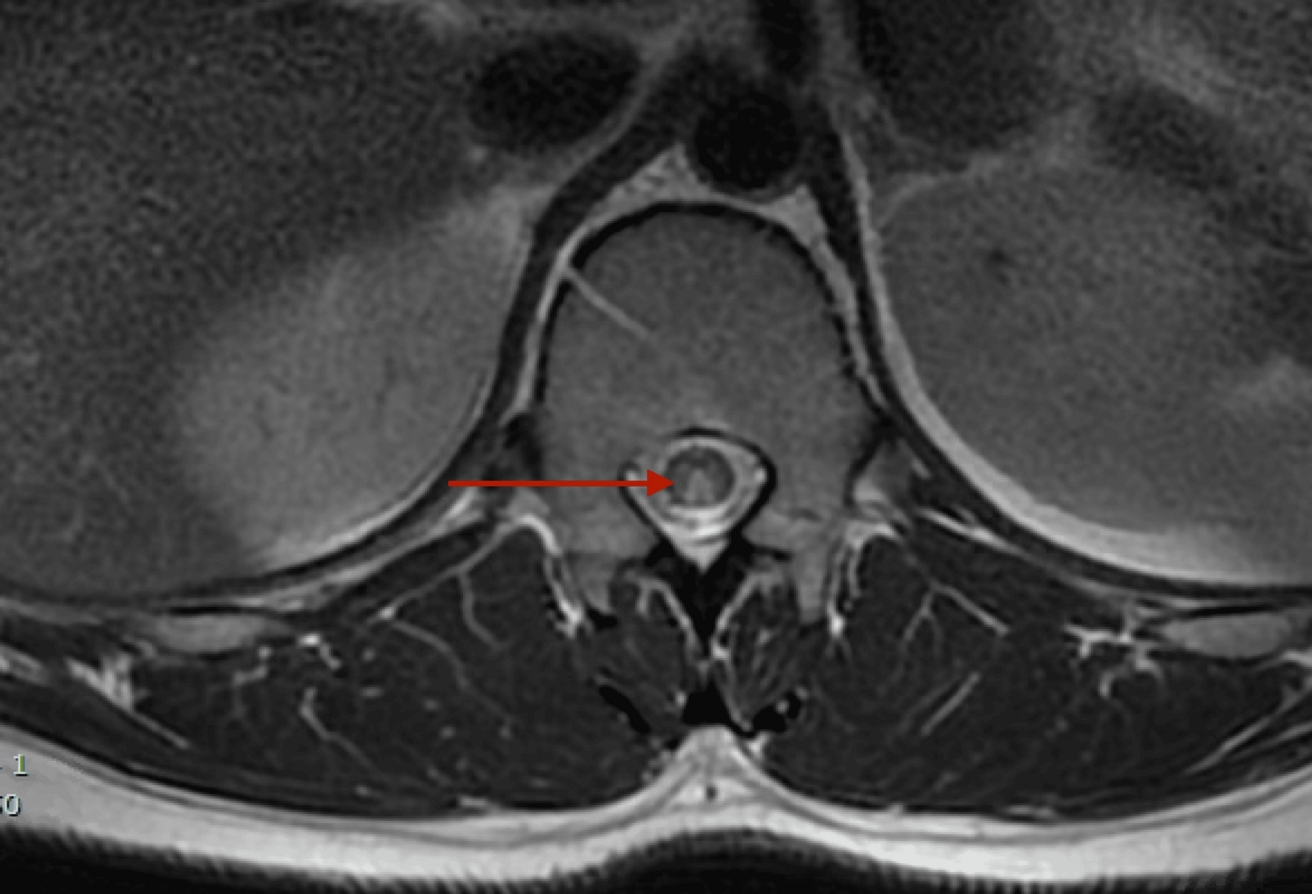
Dedicated MRI of the T-spine demonstrating long-segment subtle T2 hyperintensity within the dorsal aspect of the lower thoracic spinal cord involving the bilateral dorsal columns compatible with the diagnosis of subacute combined degeneration of the cord and may be seen in the setting of underlying metabolic abnormality such as B12 deficiency MRI: magnetic resonance imaging
